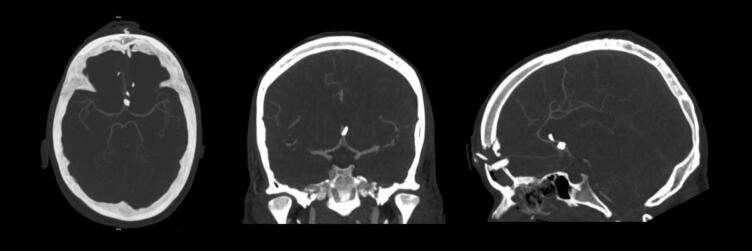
Preoperative CT brain angiogram (Axial/Coronal/Sagittal views).
